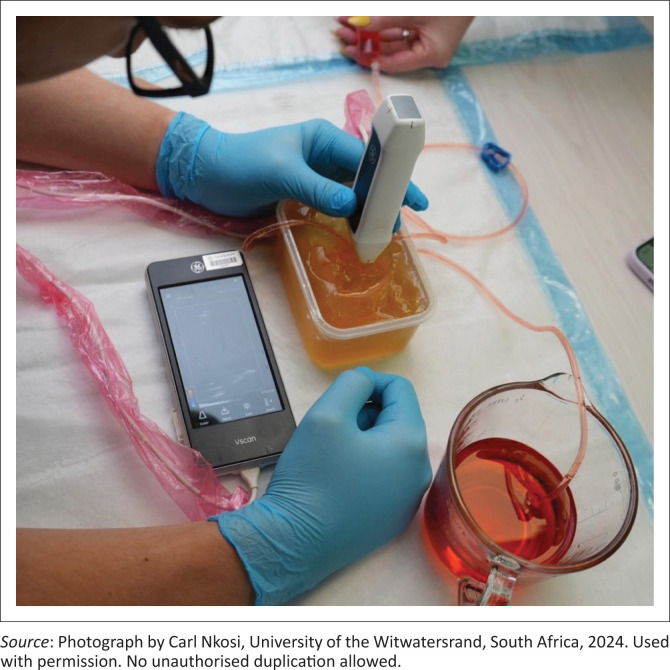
Ultrasound trainer model for demonstrating the whirlpool sign.
